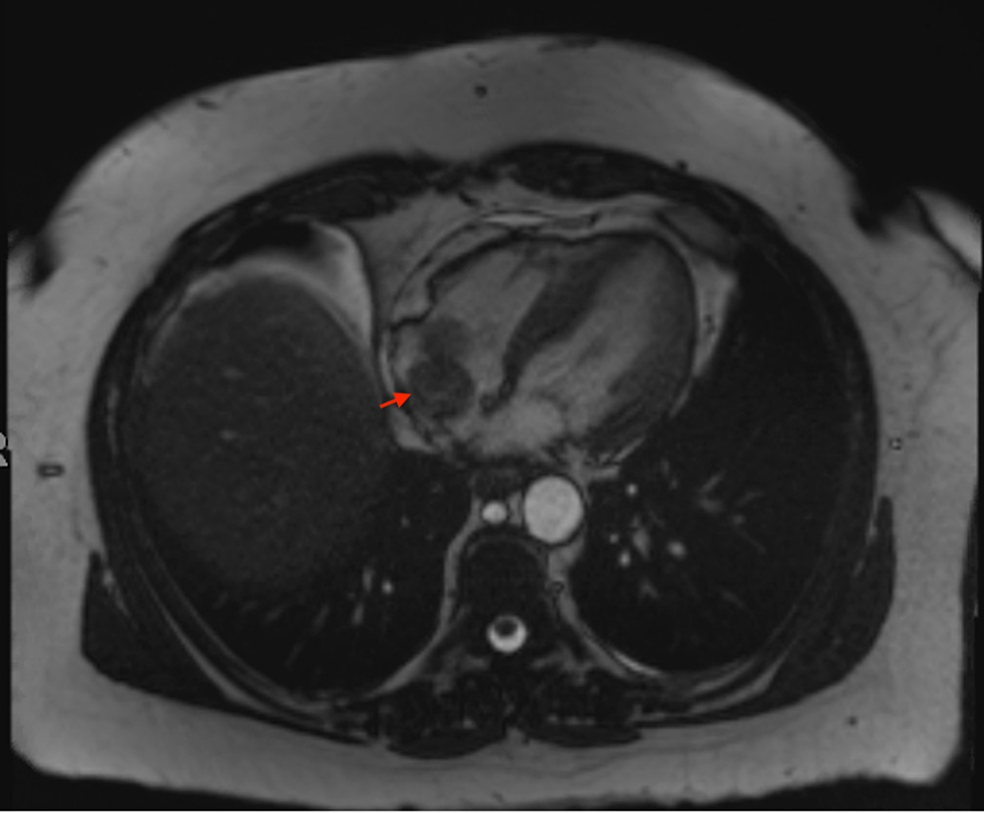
MRI of the heart with gadolinium A mobile mass is seen within the right heart, in the right atrium, and the right ventricle. The intra-cardiac portion of the mass measures 5 x 1.8 cm.
